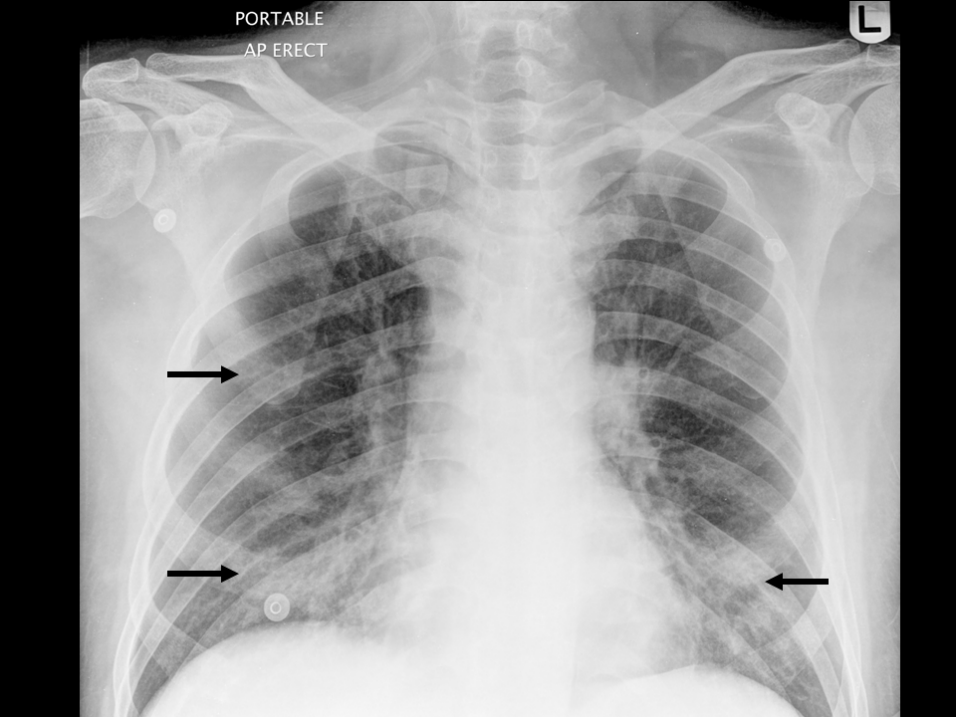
Chest X-ray with black arrows showing patchy air-space changes in both lower zones and the right upper zone.
